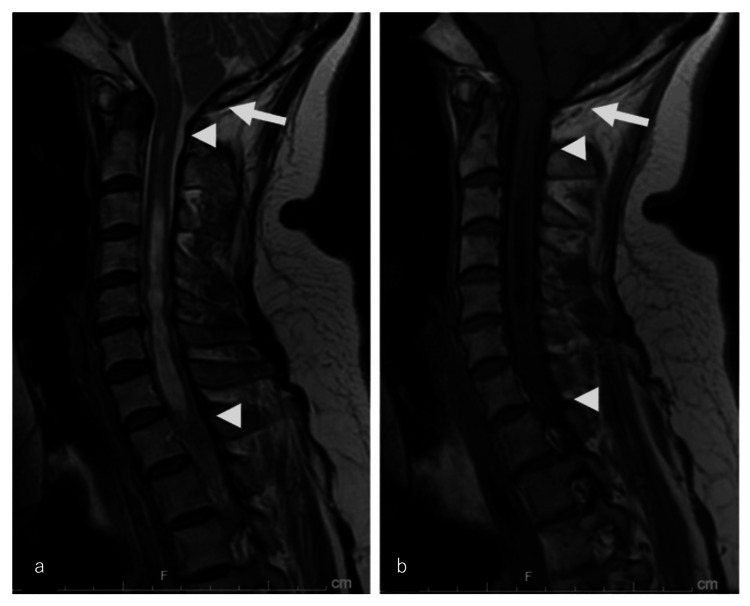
Initial cervical spine MRI obtained 21 years before presentation (a) Sagittal T2-weighted image, (b) Sagittal T1-weighted image. The arrow indicates herniation of the cerebellar tonsils below the foramen magnum. The arrowhead shows a syrinx extending from C2 to T1, appearing hyperintense on T2-weighted images and hypointense on T1-weighted images.
